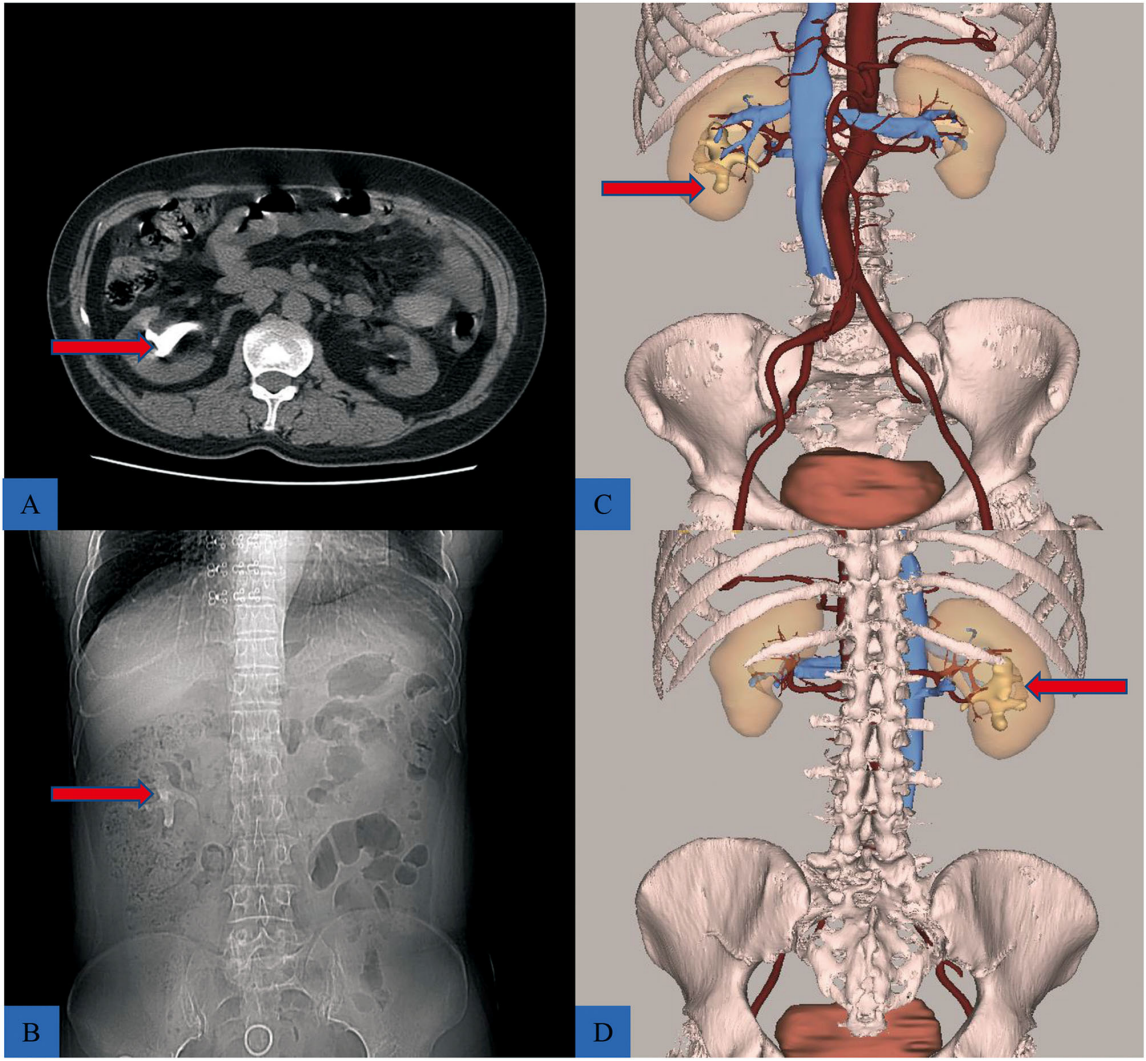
The difference between traditional CT scans, and 3D reconstructive CT scans. (A,B) The CT scan showing a staghorn stone (red arrow). (C) The front view of reconstructive urinary system including calculi (red arrow), kidney, collective system, and blood vessels, hiding the ureter to better reveal the location of the stone. (D) Posterior view of the puncture location, during PCNL.
